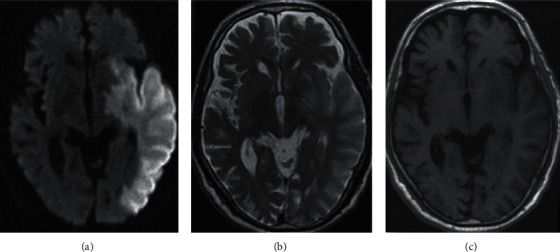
Comparison of MRI images of stroke patients under different noise intensities. (a) 5%, (b) 1%, and (c) noise-free MRI images.
